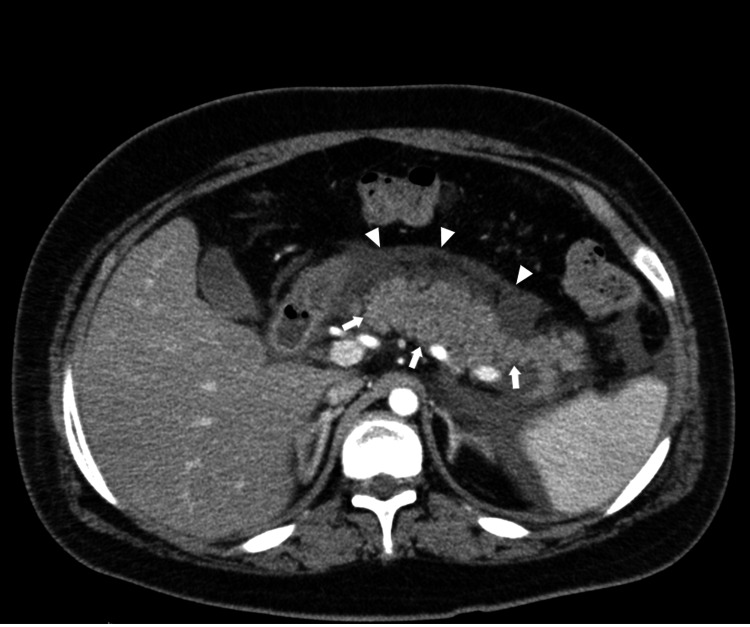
Arterial phase abdominal dynamic CT showing pancreatic enlargement (white arrow), an enhanced density of retroperitoneal adipose tissue, and retroperitoneal fluid retention (white arrow heads) CT showed no areas of poor contrast in the pancreatic parenchyma, dilatation of the main pancreatic duct, or calcified stones.
